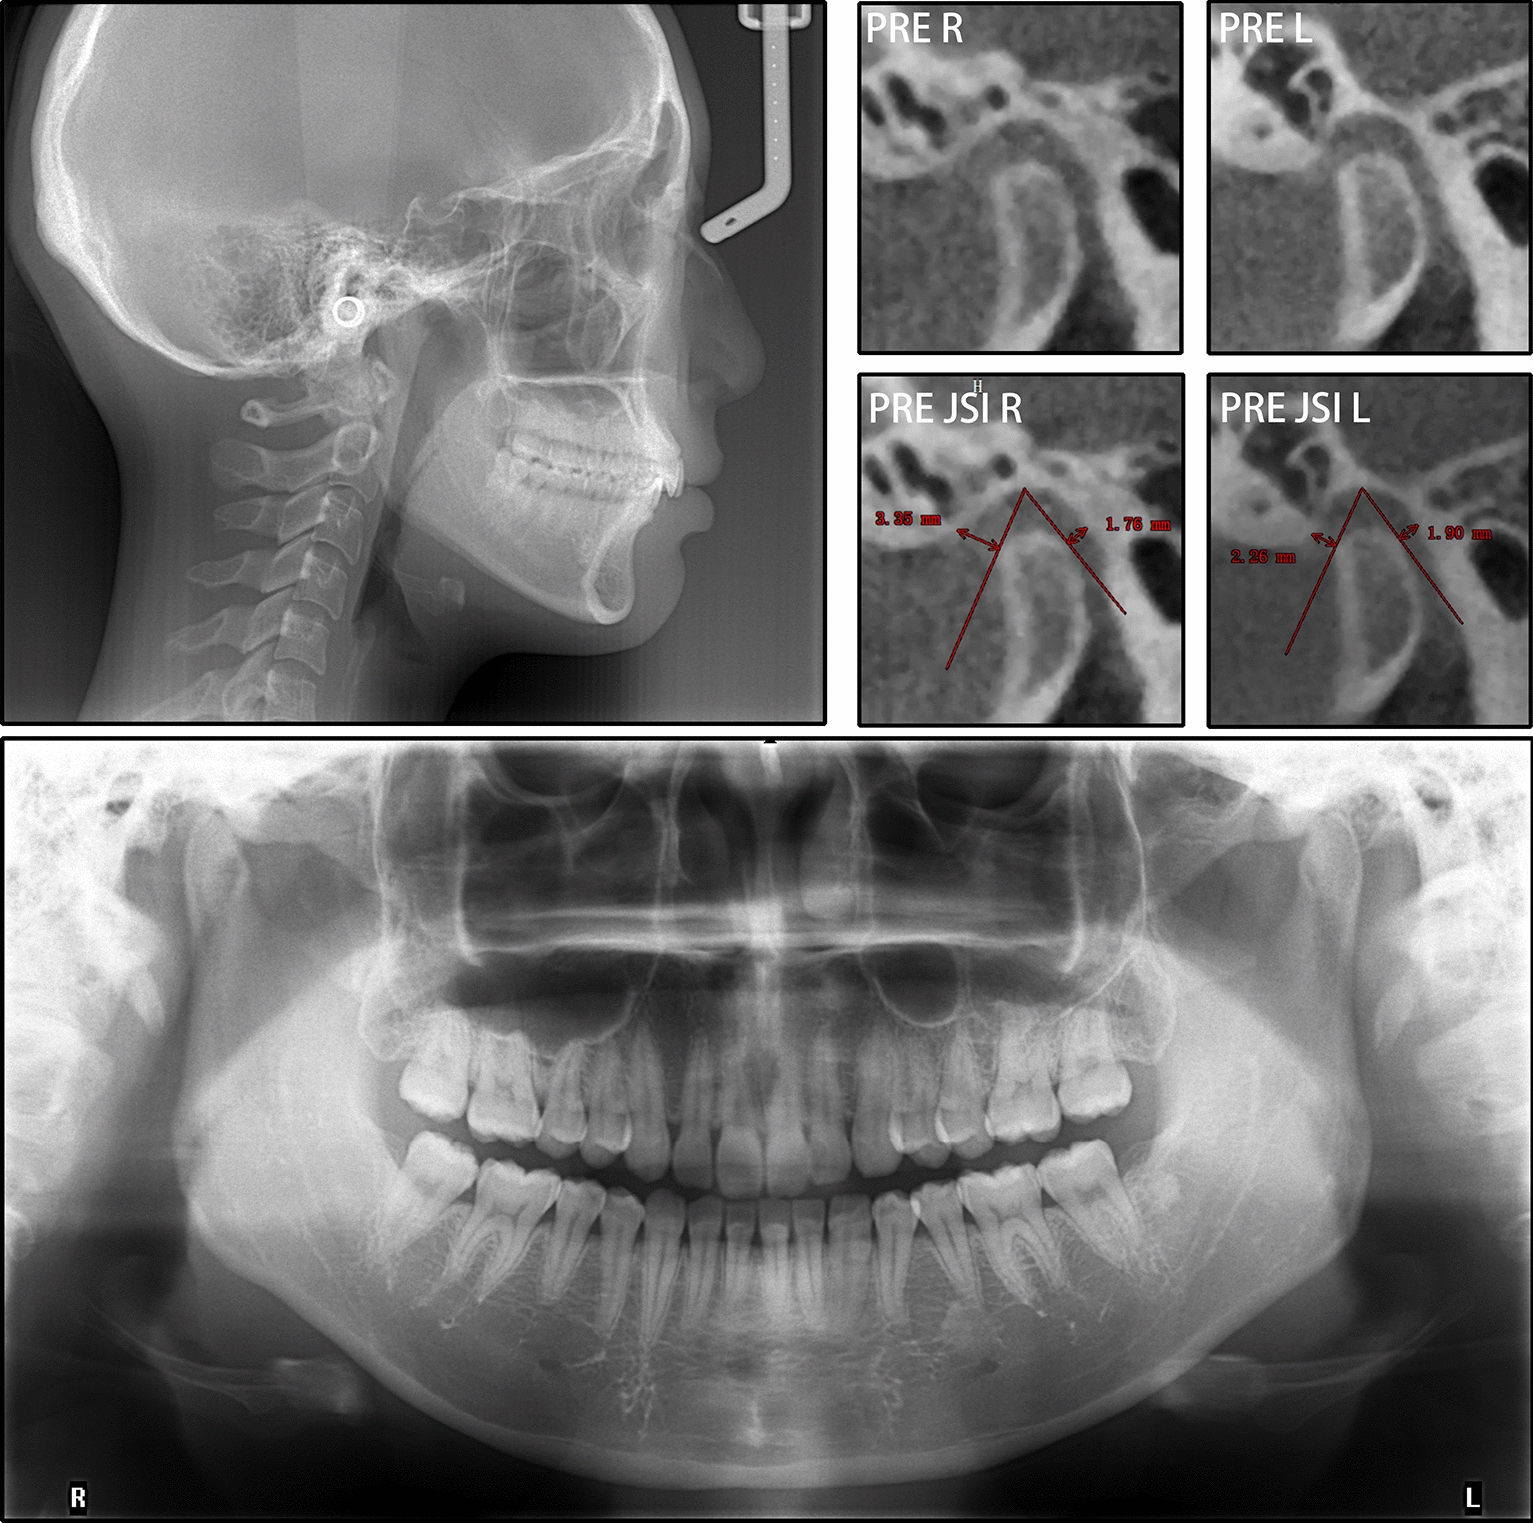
Pretreatment radiographs and bilateral TMJ JSIs
